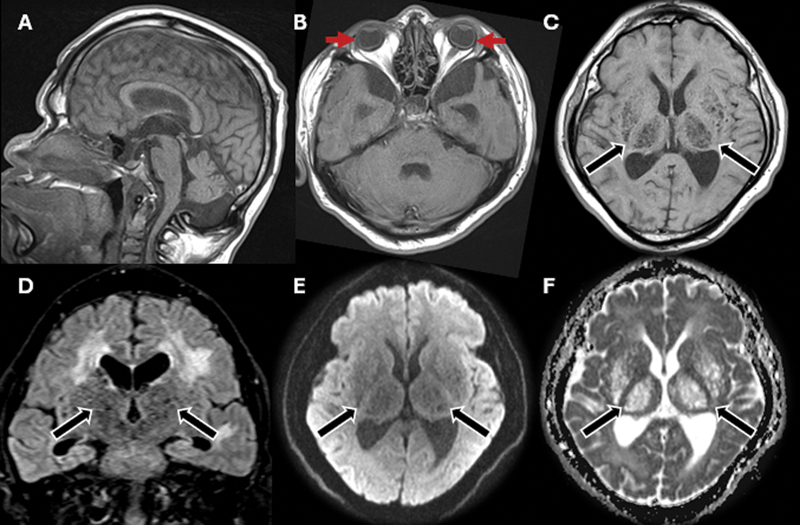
Brain MRI scan, including sagittal T1-weighted (
